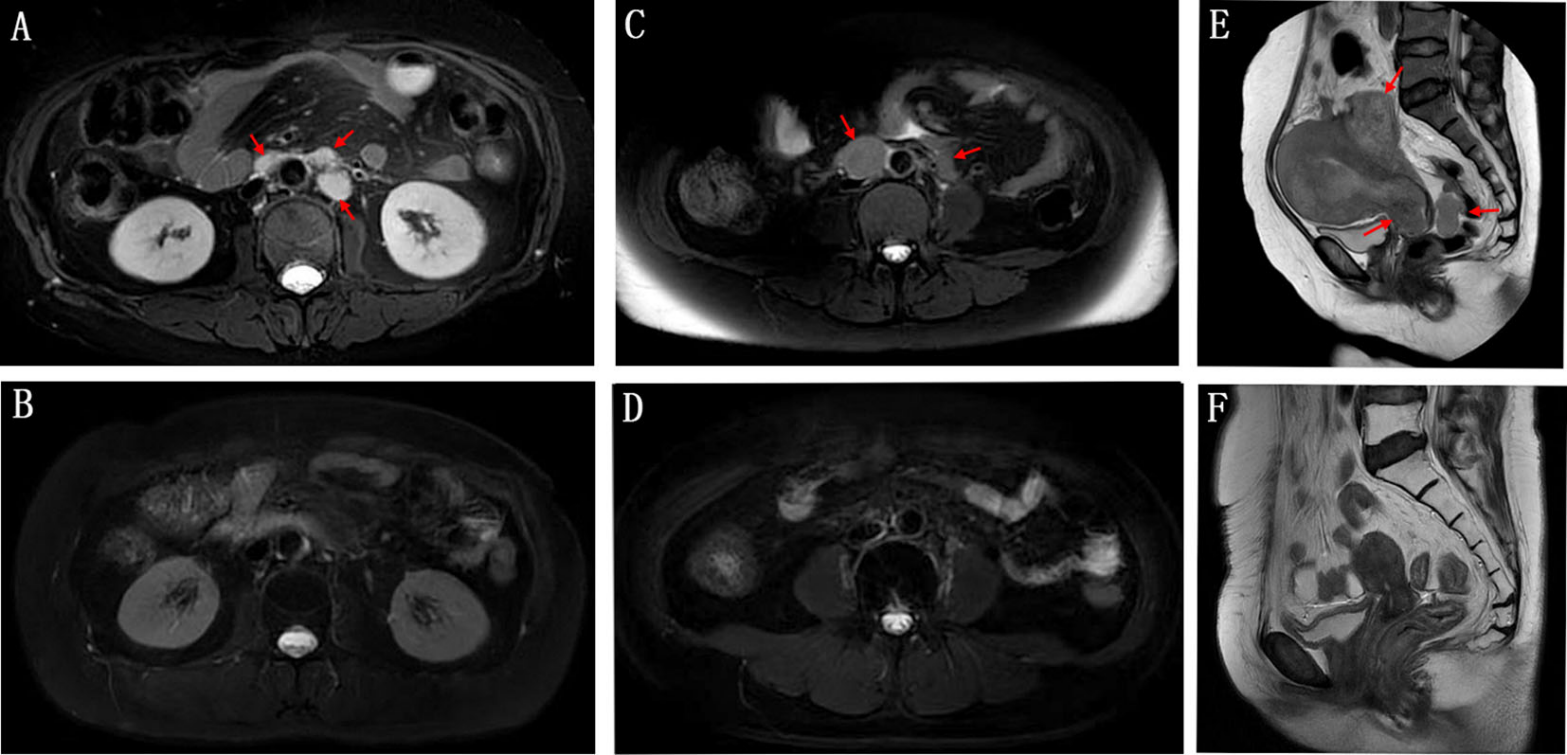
Magnetic resonance imaging (MRI) findings of a patient initially dosed in March 2023 achieved CR (complete remission) in February 2024 after 15 cycles of treatment. The patient’s condition remained stable until the end of the last follow-up. The upper panel shows the patient’s retroperitoneal metastatic lymph nodes (A), pelvic metastatic lymph nodes (C), and pelvic lesions (E) before treatment. (B, D, F) shows the changes after treatment.
